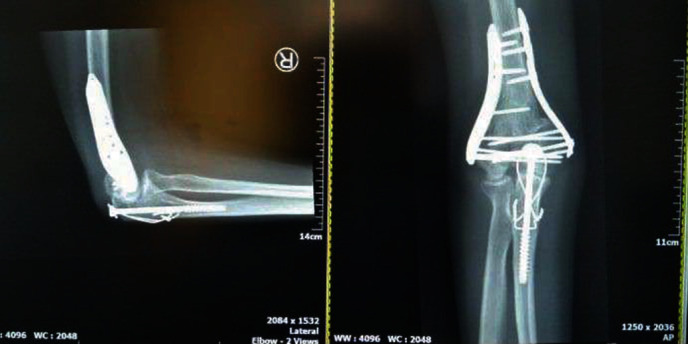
X-rays of the elbow six months postoperation.
